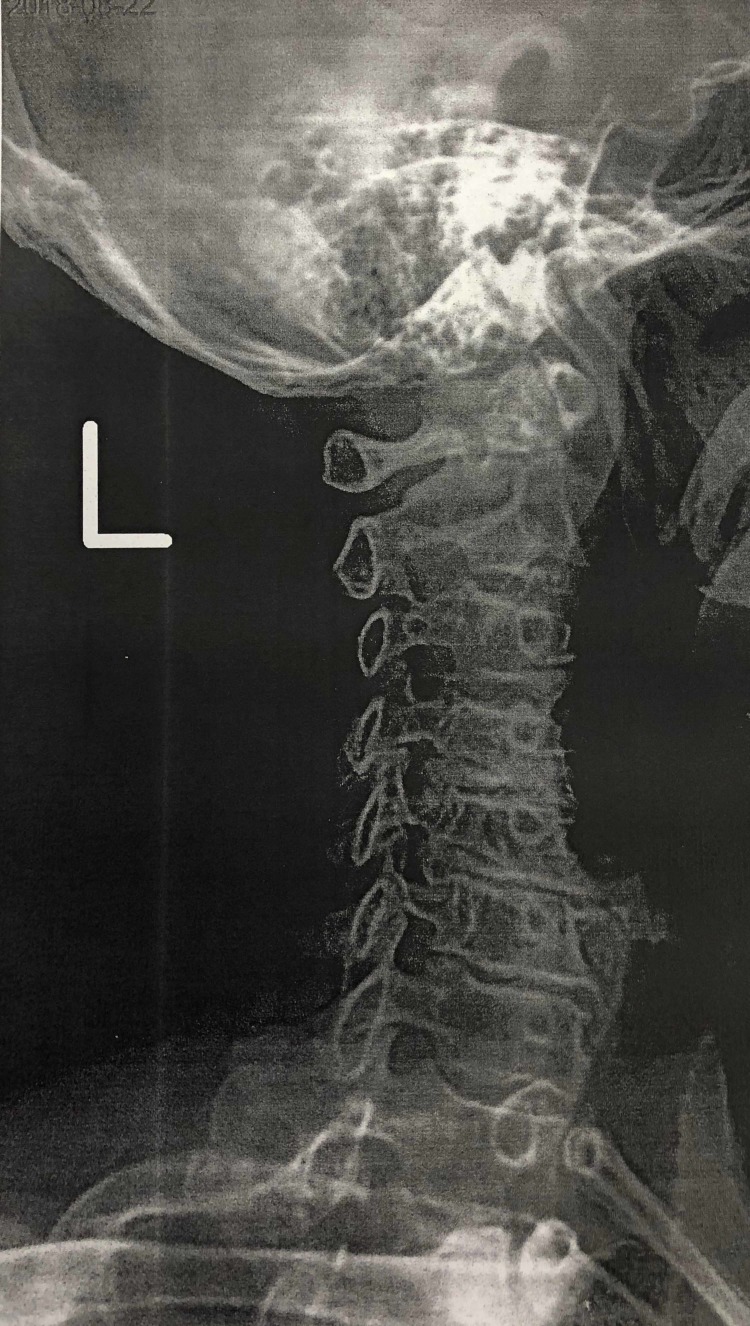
Left cervical spine x-ray demonstrating moderate to severe degenerative changes at the level of C4-C7 with intervertebral foramen narrowing, consistent with symptomatic cervical spine disease.
